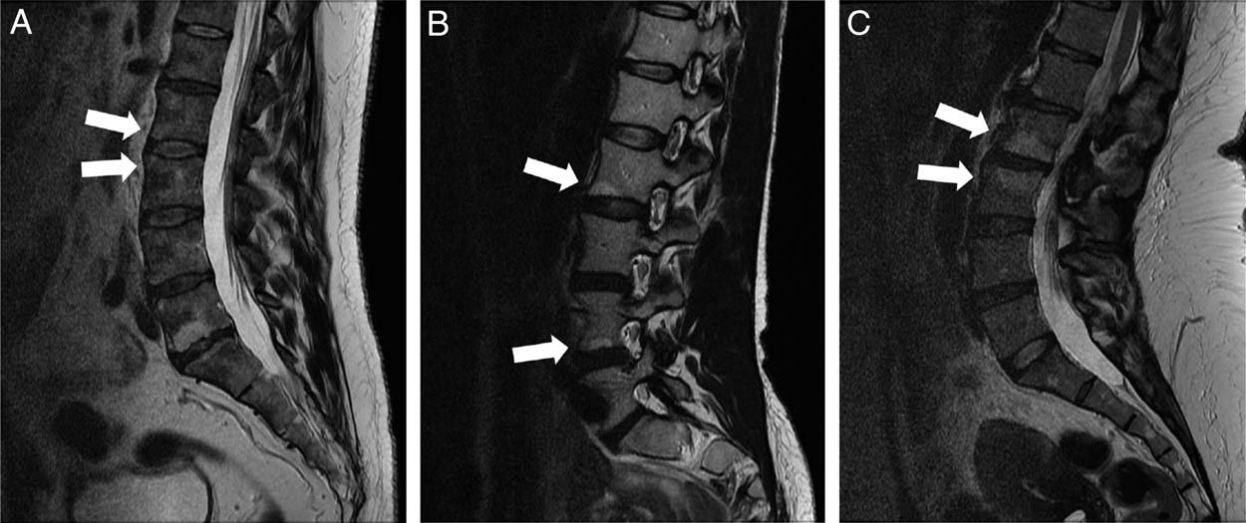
Lumbar T2 magnetic resonance imaging slices showing cases of disagreement on Modic change classification between SpineNet and Finnish radiologists. A, Mid-sagittal slice of L2-L3 intervertebral disk with upper and lower end plates coded as having Modic changes present by SpineNet but coded as having no Modic changes by radiologists. B, Lateral sagittal slice of L2-L3 and L4-L5 intervertebral disks with upper end plates coded as having no Modic changes by SpineNet but coded as having Modic changes by radiologists. C, Mid-sagittal slice of L2-L3 intervertebral disk upper and low end plates coded as having no Modic changes by SpineNet but coded as having Modic changes by radiologists.
